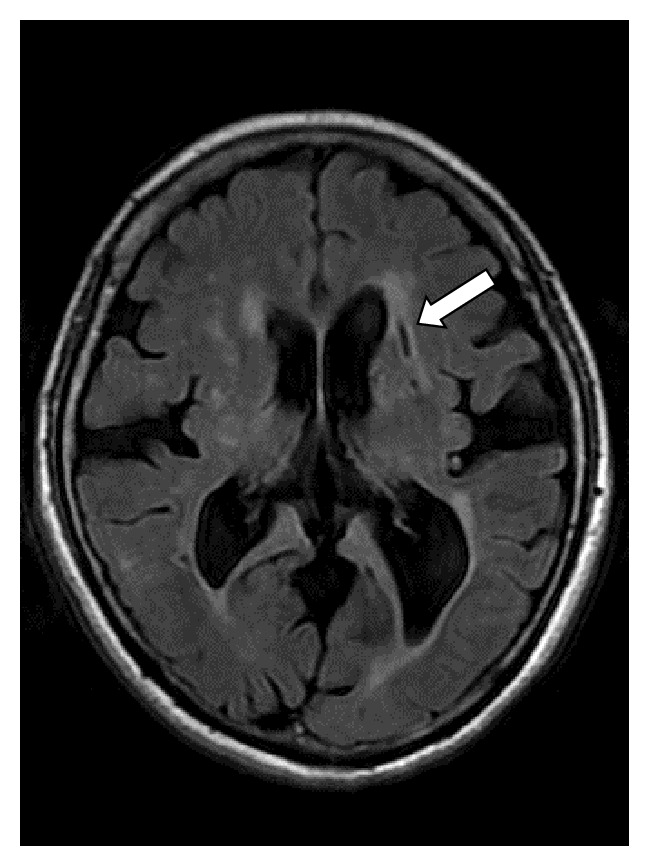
A 60-year-old female patient with type 2 diabetes mellitus for 12 years with lacunar brain infarcts (arrow) on a FLAIR sequence and aortic arch PWV of 10.56 m/s and brachial artery FMD of 13.64%.
